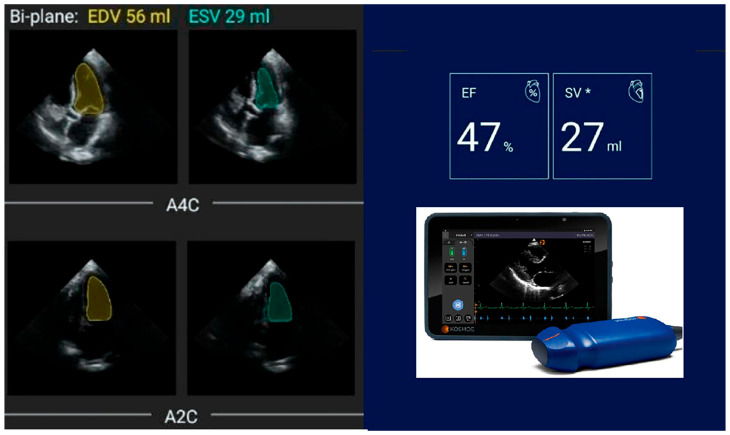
Following the recommendations of the American Society of Echocardiography and the European Association of Cardiovascular Imaging (modified Simpson’s rule), AI algorithm first identifies end-systolic (ED) and end-diastolic (ES) frames in the A4C and A2C clips, then segments LV in all four frames (A4C ED and ES frames, A2C ED and ES frames) to automatically calculate LVEF in just 5 s. * Stroke Volume.
